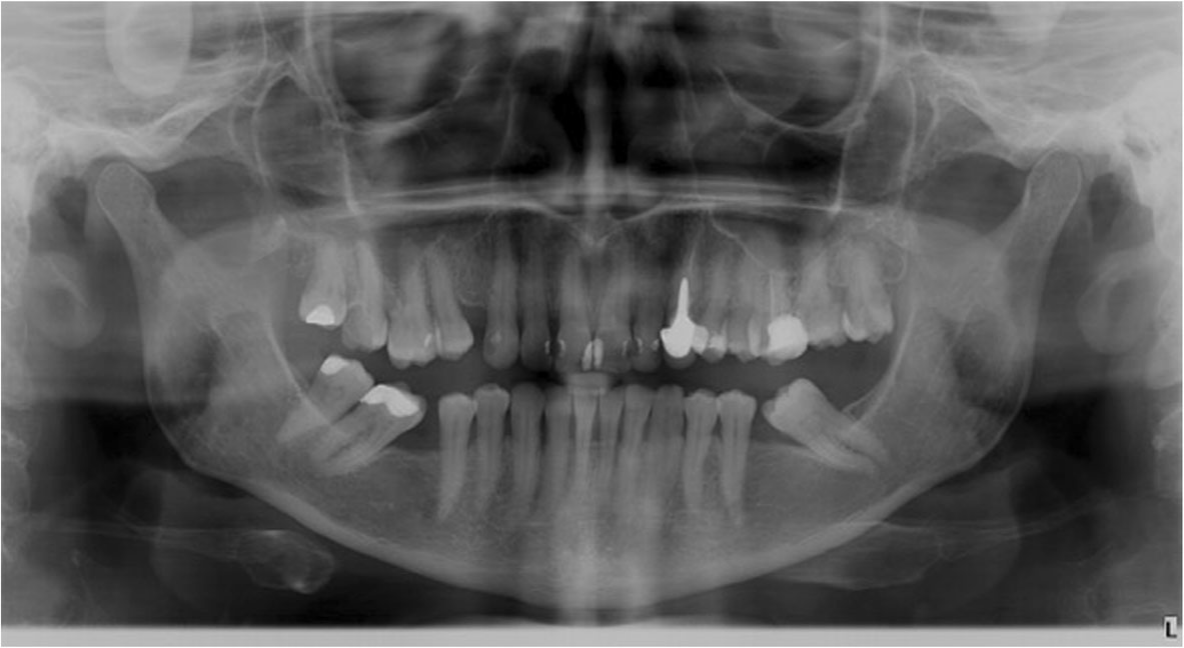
Panoramic radiograph with C2 category of MCI
